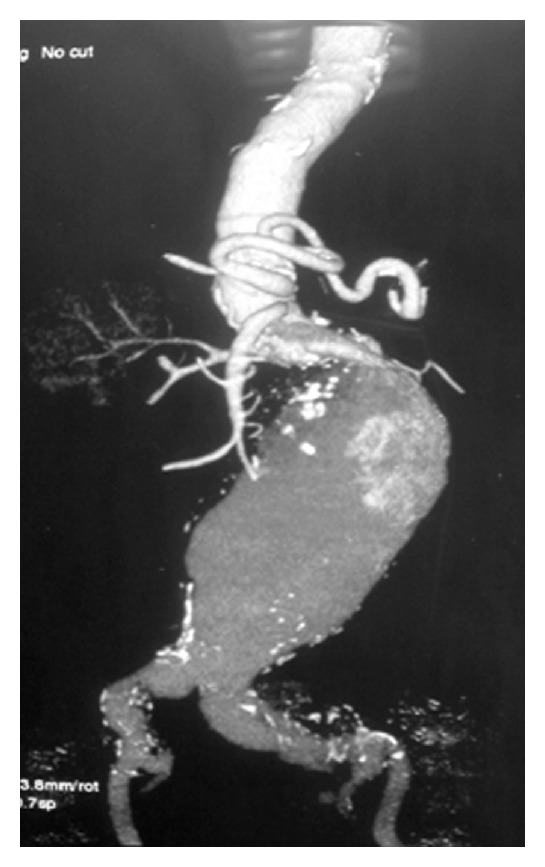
CT angiography of the anatomy of the AAA.
